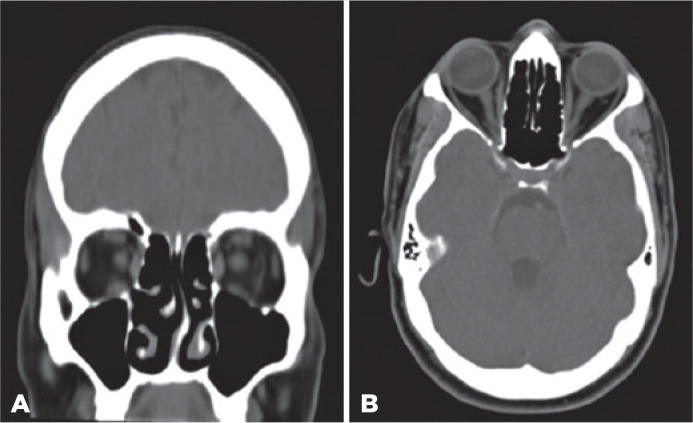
Head CT scan without contrast. Neuroimaging evaluation in a 44-year-old
